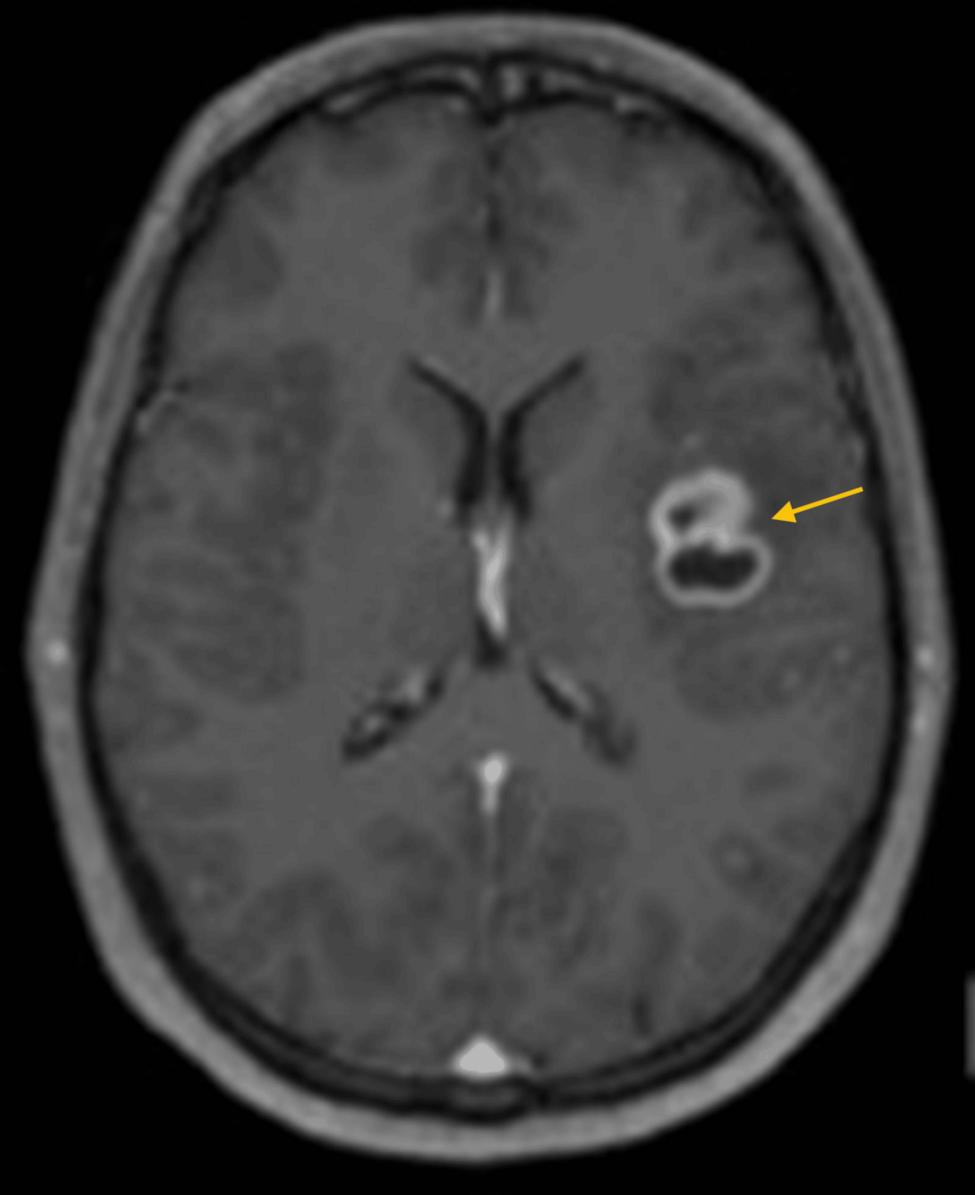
Pre-operative MRI (T1 with gadolinium contrast) The yellow arrow highlights a parenchymal lesion in the left fronto-insular region with contrast enhancement and central necrotic/cystic core, suggestive of a high-grade glioma.
